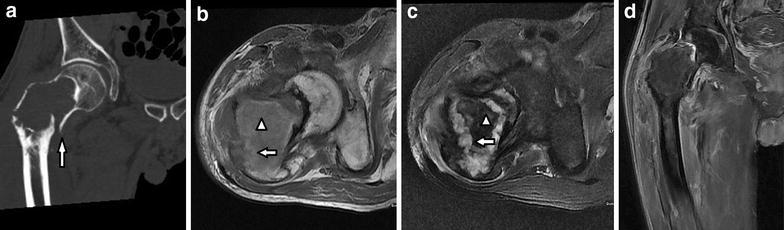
Case 18: a Coronal CT image shows a pathological fracture (*white arrow*) of the neck of femur secondary to an osteolytic destructive lesion with soft-tissue mass. b, c Axial T1-weighted and fat-supressed T2-weighted MR images show a large heterogeneous mass and hypointense and hyperintense on T2WI in the centre of the lesion are suggestive of hemorrhage (*arrowhead*) and necrosis (*white arrow*) respectively. d Coronal fat-supressed T1-weighted postcontrast image shows that the lesion has an enhancement in the surrounding soft tissue
